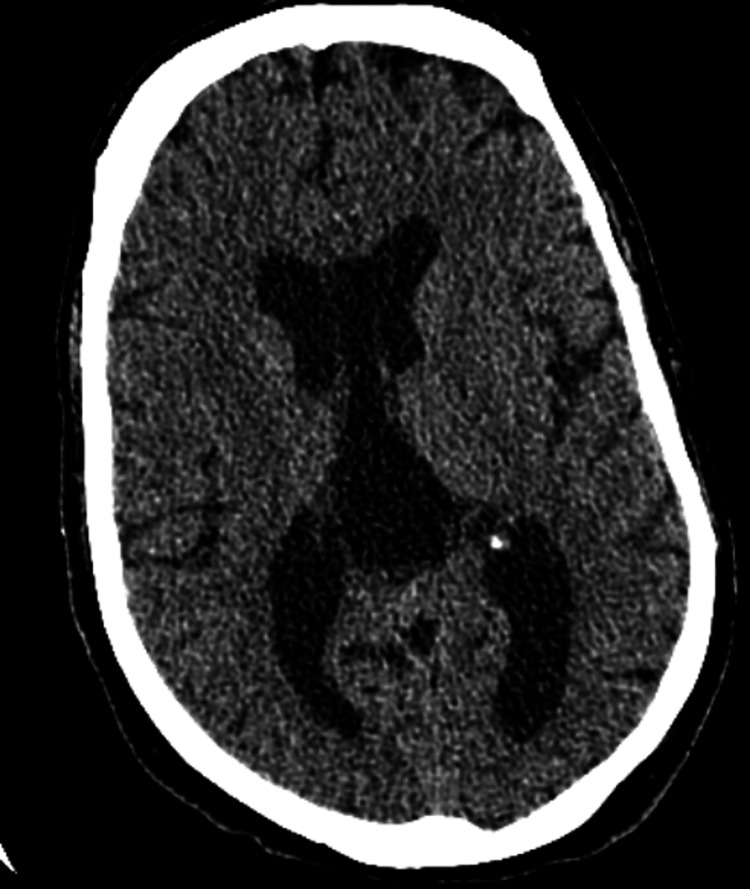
CT scan of the head without contrast negative for any infarct, hemorrhage, or mass effect
